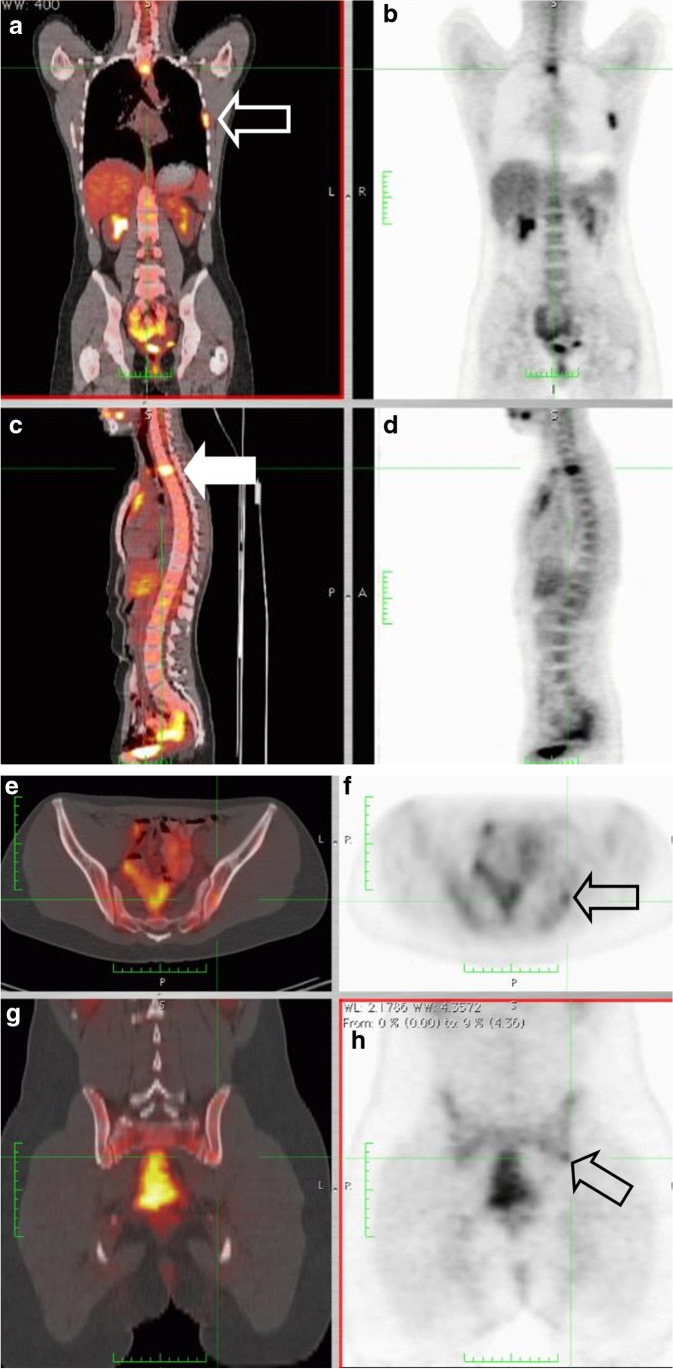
a–h Coronal (a, b, g, h), sagittal (c, d) and axial (e, f) fluorodeoxyglucose-positron emission-tomography-computed tomography images in 15-year-old girl (patient 2) approximately 17 months after completion of chemotherapy show multiple metastases with increased tracer uptake of the left 3rd rib (a, b) (*arrow* in a), the 12th thoracic vertebral body (c, d) (*arrow* in c) and osseous metastases in the pelvis (e–h) (*arrows* in f and h)
